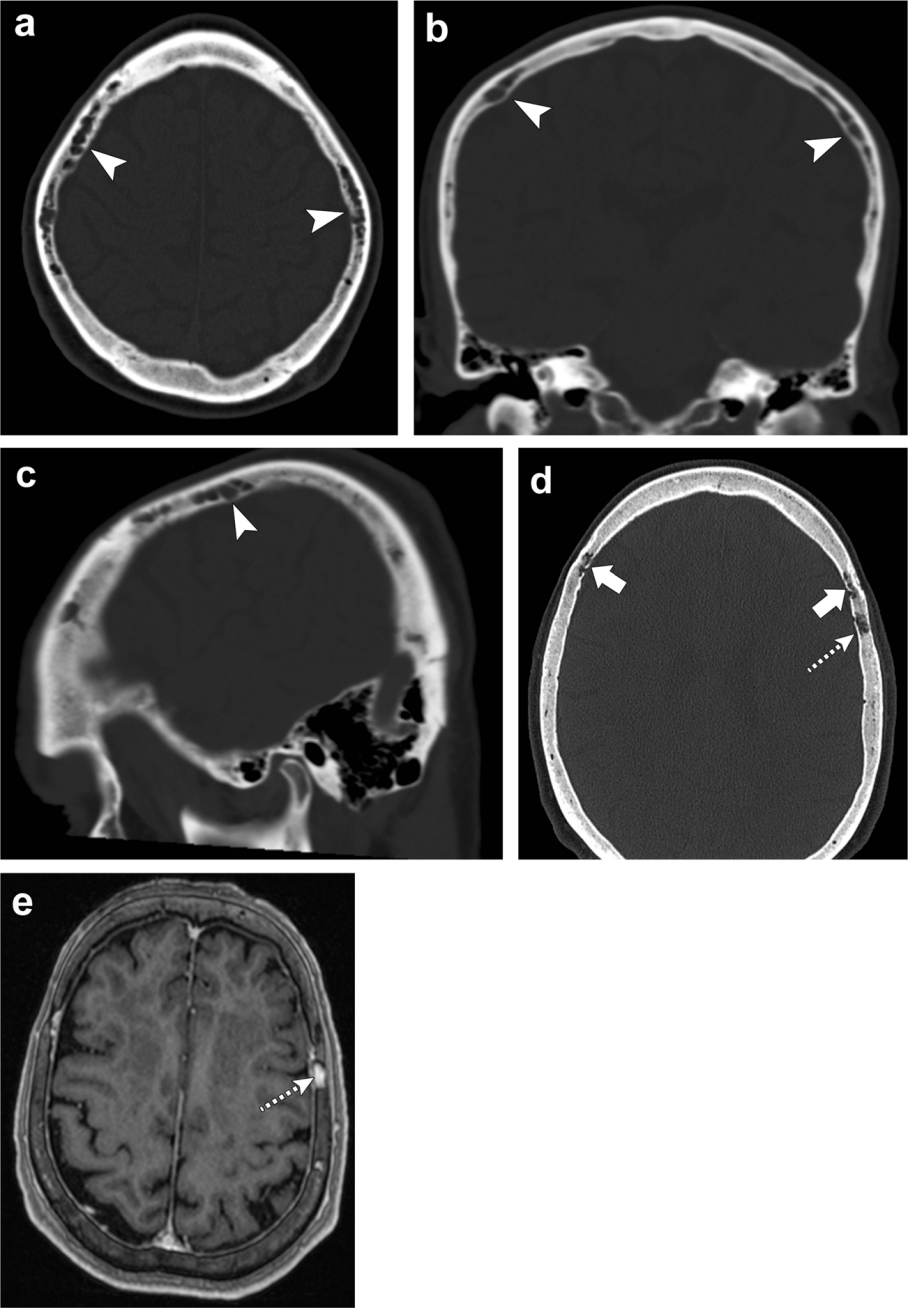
Venous channels and lakes. Axial (a), coronal (b) and sagittal (c) head CT images depict serpiginous structures in the frontal and parietal bones (*arrowheads*) representing prominent venous channels. Axial head CT (d) and axial post-contrast T1-weighted (e) images show a lucent focus along the left parietal bone with enhancement consistent with a venous lake (*dashed arrows*). There are also mildly prominent serpiginous venous channels in the frontal bones (*thick arrows*)
